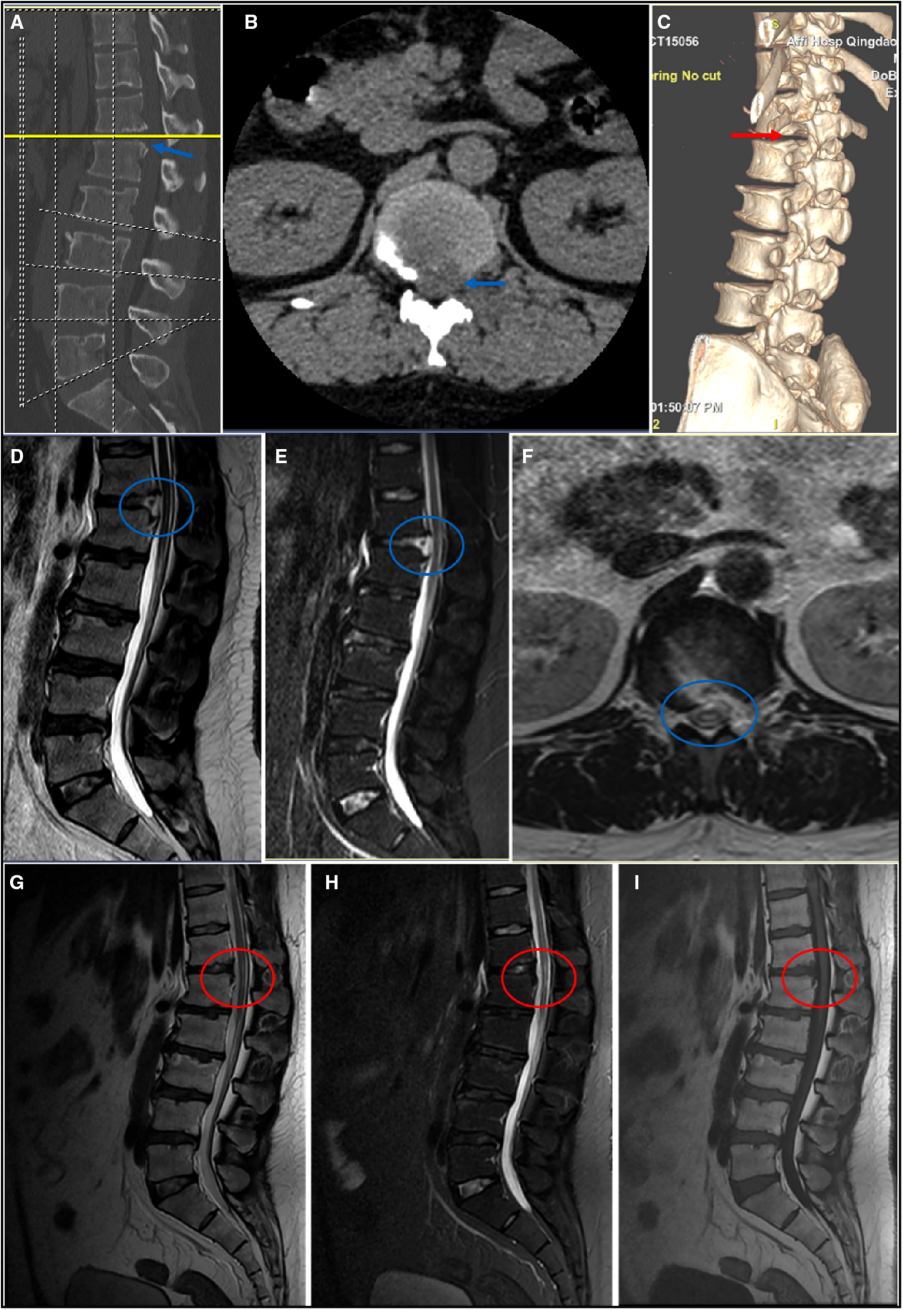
Postoperative imaging of the typical patient with disc herniation at the T12–L1 level. (A–C) CT at 1 day postoperatively. The blue arrow shows sufficient removal of calcific herniated disc. The red arrow shows that the SAP is partly resected and the stability of the spine is preserved. (D–F) MRI at 1 day postoperatively. The blue circle shows complete decompression of dura sac with a small amount of fluid signal in the ventral side of dura sac. (G–I) MRI at 6 months postoperatively. The red circle shows perfect decompression of dura sac and normal signal of cerebrospinal fluid surrounding the dura. (A,D,E,G–I) sagittal view; (B,F) axial view; (C) three-dimensional reconstruction. SAP, superior articular process; CT, computed tomography; MRI, magnetic resonance imaging.
